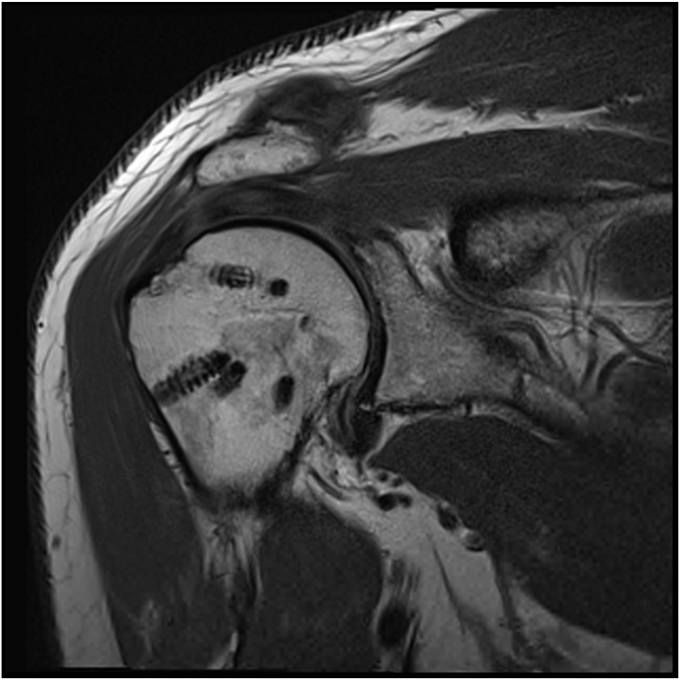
Healed massive cuff repair (Sugaya grade I) of patient 11. MRI performed 6 months after surgery.
